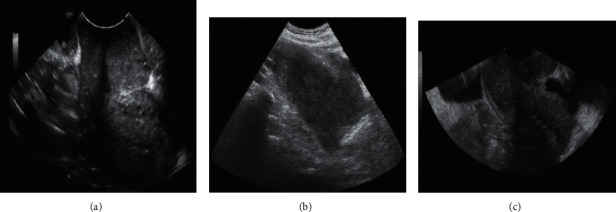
Traditional ultrasound image of cervical cancer. (a) An image of the early symptoms of cervical cancer. (b) The uterine cavity is closed and the periphery of the cervical lesion is blurred. (c) The cervical lesions are visible.
